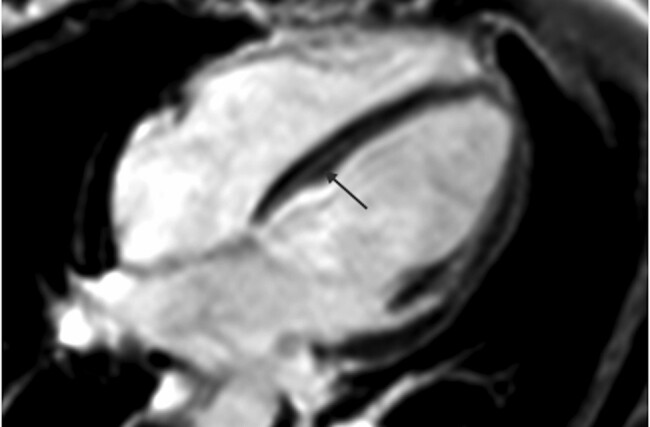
Cardiac magnetic resonance imaging of Case 3. A four-chamber view with contrast showing late gadolinium enhancement of the midwall from basal to midseptum as see by arrow.
